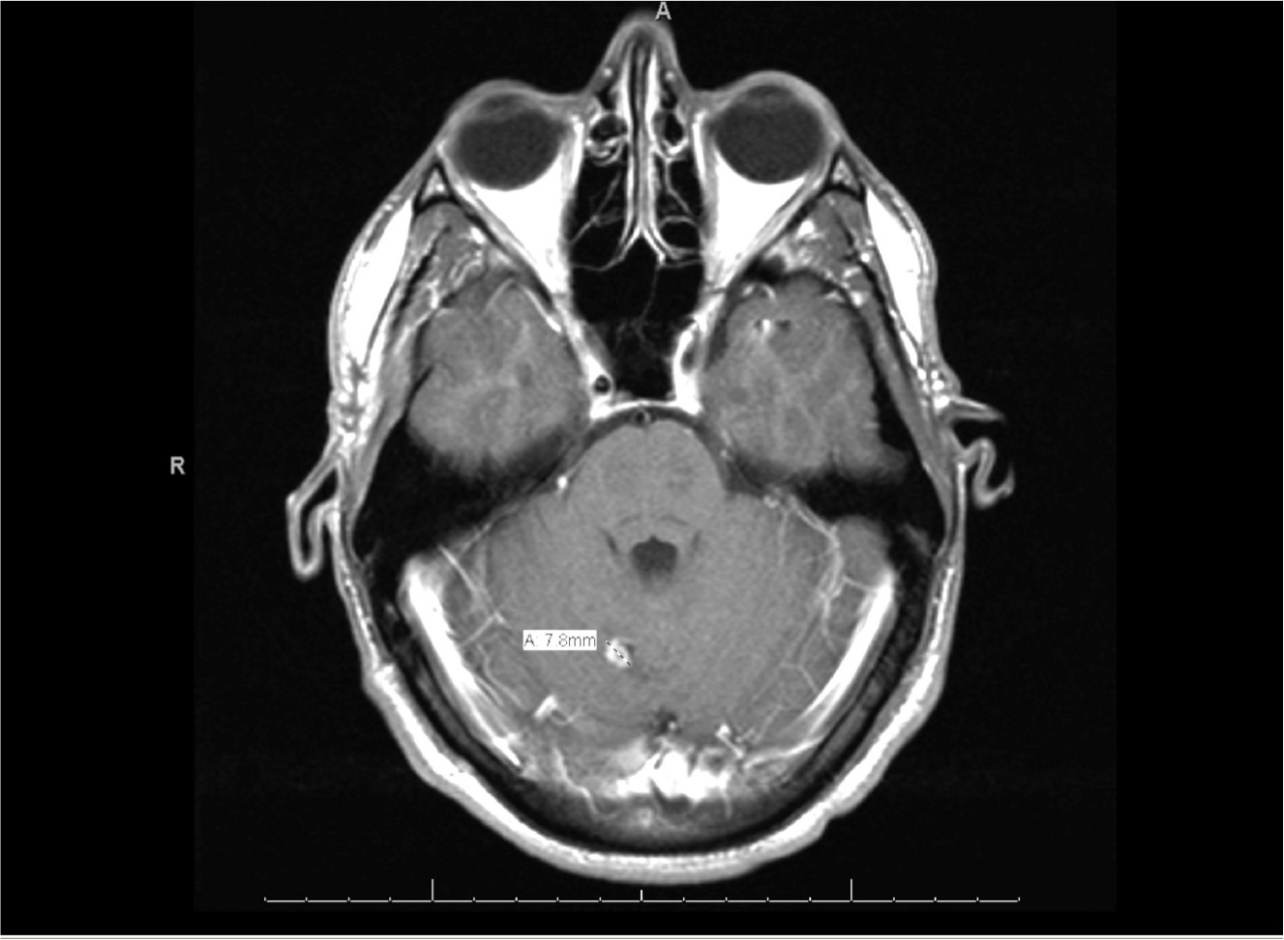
MRI One Month Post-Resection New definite enhancing foci compatible with metastatic foci.
